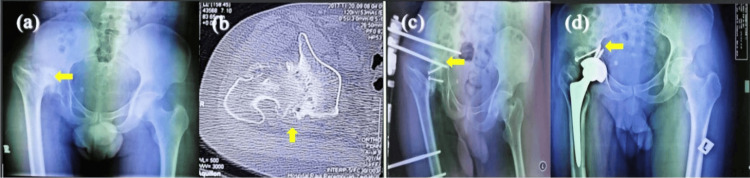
Childhood hip deformity with limb length discrepancy > 7 cm These are the x-rays of a 31-year-old male. (a) Preop: Proximal migration of femur with acetabular defect and pelvic tilted. Arrow showed shallow acetabulum with small femoral head. (b) Axial CT scan shows femoral head deformity with acetabular defect (arrow). (c) First stage procedure by using femoral head autograft for acetabular reconstruction. Arrow showed acetabular defect at superolateral reconstructed use small femoral head. (d) Second stage procedure: Total hip replacement after removal intra acetabular fibrosis. Interval between first and second stage was 60 days. Arrow showed good graft incorporation.
